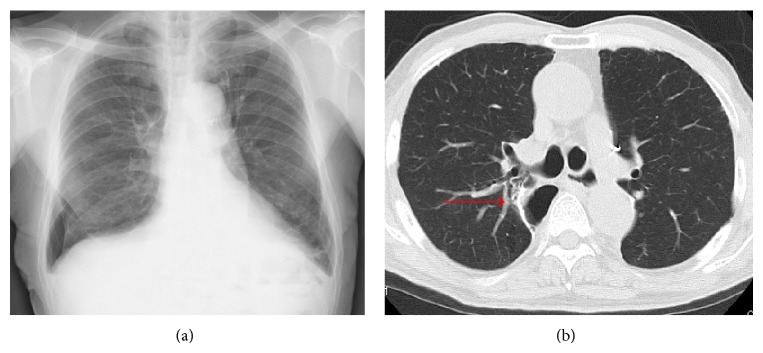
(a) A right pneumothorax is observed in the lower right lung field. (b) The thoracic CT findings suggest that the fistula might exist in the periphery of the B^2^a bronchus (arrow).
